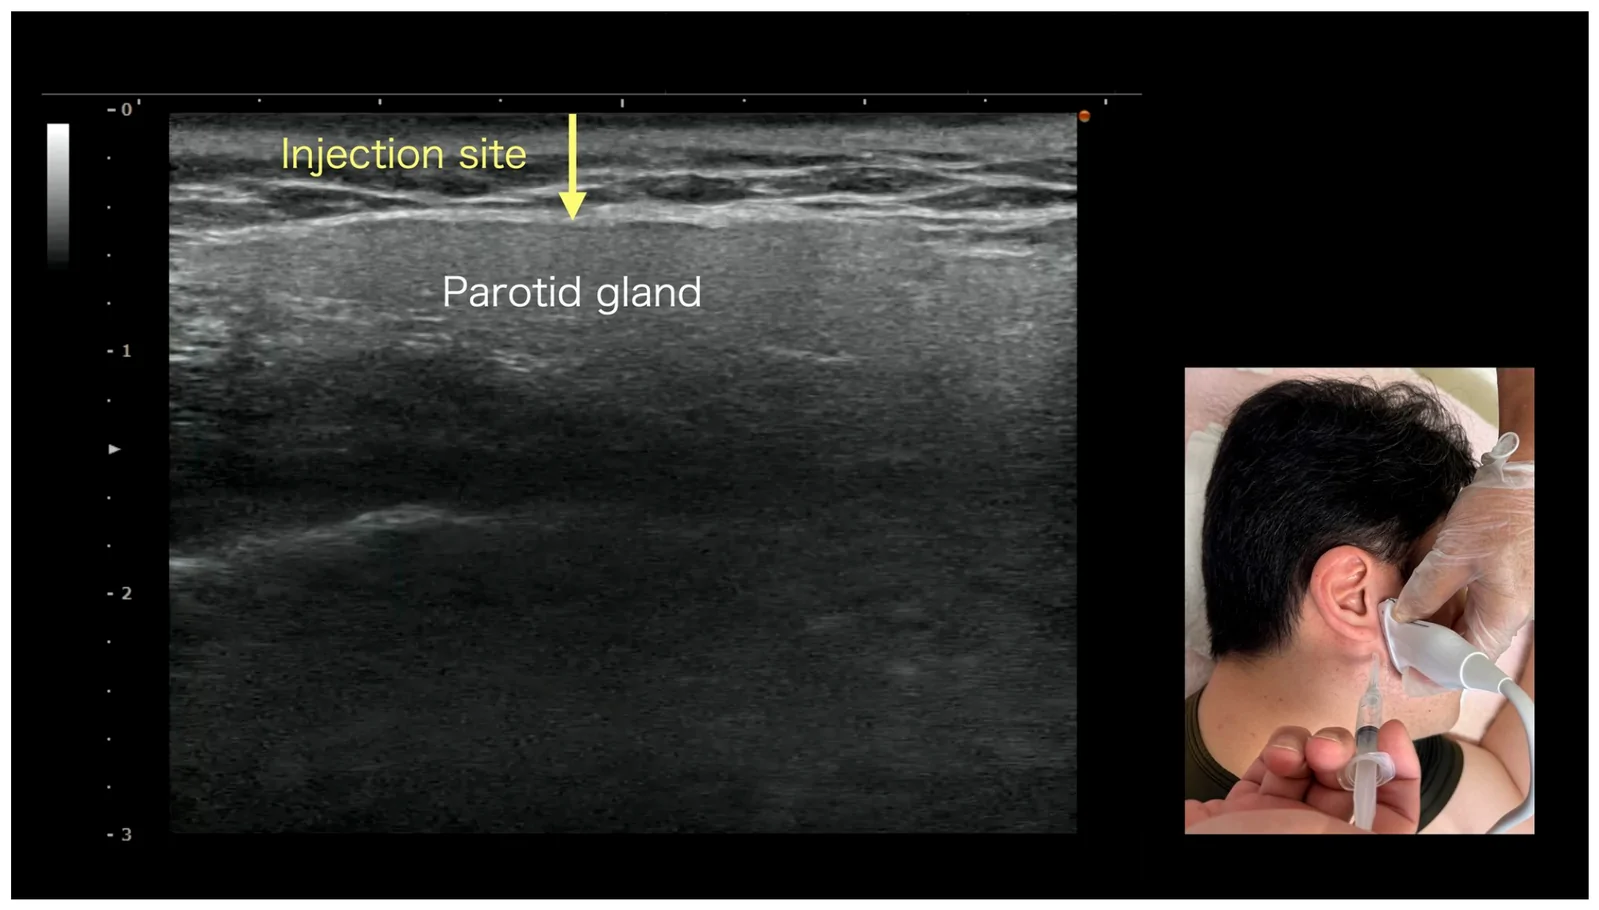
Superficial parotid fascia US-FHR (POINT 7). Ultrasound image: The parotid gland is identified. The yellow arrow (injection site) points to the densified area of the superficial parotid fascia. With a 30 G needle, 0.5–1 mL of saline is injected via the out-of-plane approach. The needle tip is kept superficial to the parotid gland capsule. The inset (bottom right) shows the patient position and probe placement.
